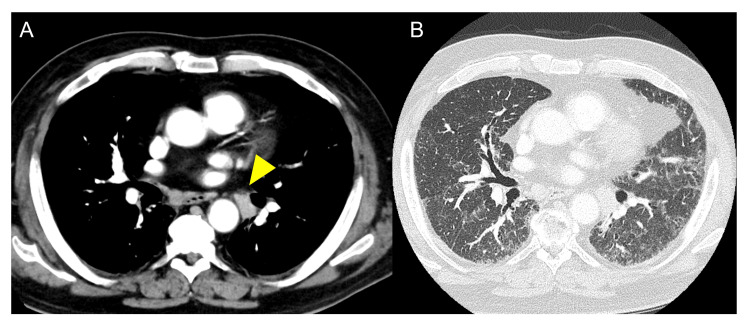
Image of the first admission to our institution. (A) and (B) Contrast-enhanced computed tomography on admission showing enlarged lymph nodes (yellow arrow).
